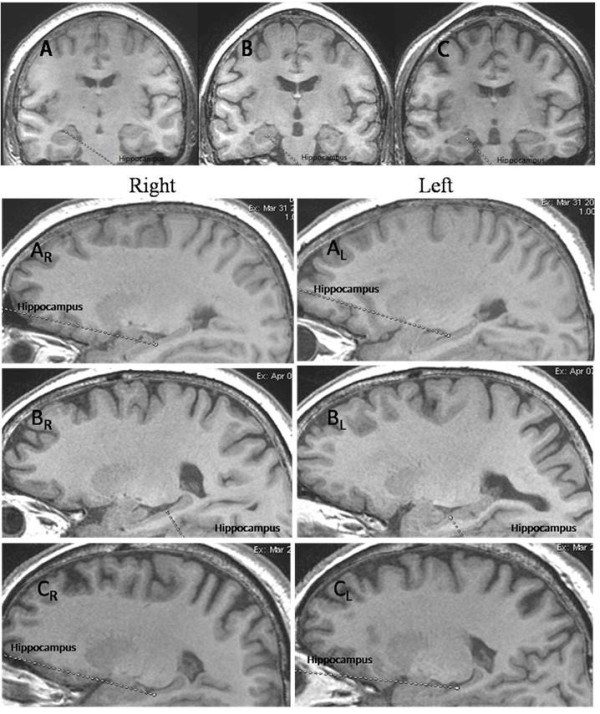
MRI images from a control subject, a mild-to-moderate COPD patient and a severe COPD patient. A line has been placed over the region of the hippocampus in one hemisphere. A = control subject; B = mild-to-moderate COPD patient; C = severe COPD patient; A_R_ = the right hippocampus of control subject; A_L_ = the left hippocampus of control subject; B_R_ = the right hippocampus of mild-to-moderate COPD patient; B_L_ = the left hippocampus of mild-to-moderate COPD patient; C_R_ = the right hippocampus of severe COPD patient; C_L_ = the left hippocampus of severe COPD patient.
